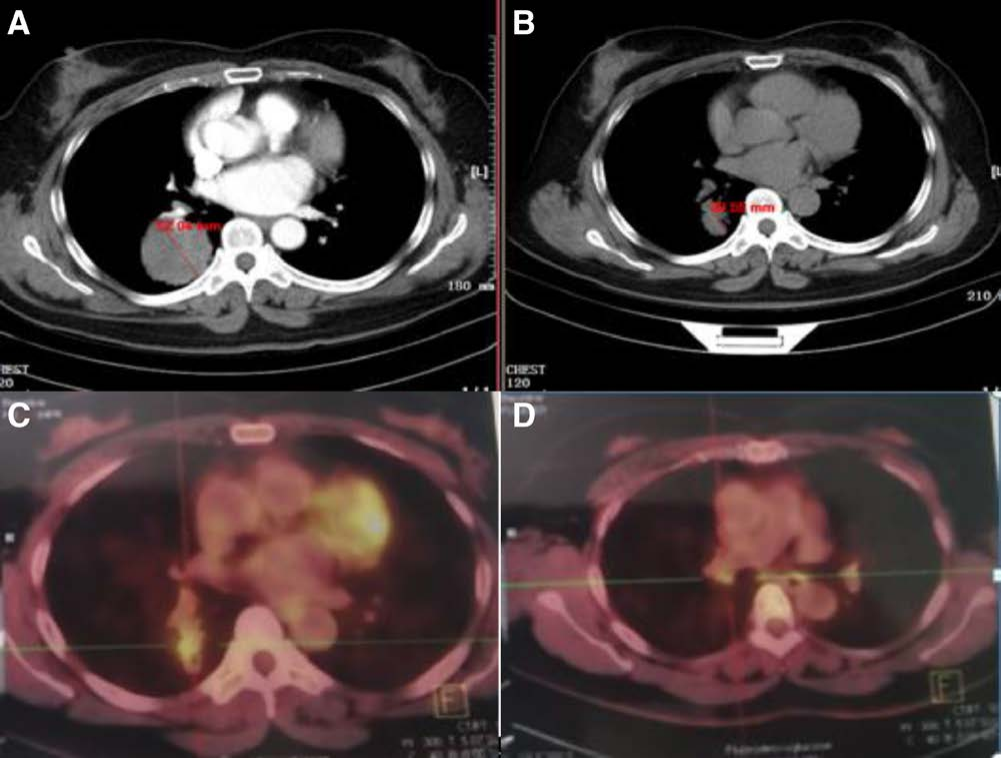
Radiological images (computed tomography and 18F-fluorodeoxyglucose/positron emission tomography), showing the changes of lung masses during treatment course. Pretreatment (A), one-month treatment (B) and 3-month treatment (C and D) with neoadjuvant icotinib.
